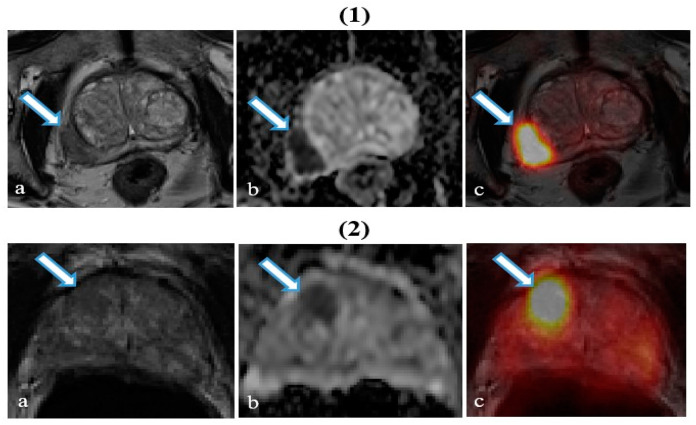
Patients with prostate cancer to the left of the peripheral zone with a Gleason score 4 + 5 = 9 (1) and transitional zone with a Gleason score 3 + 4 = 7 (2). The arrows in the axial section point to areas of cancer. (a) T2-weighted image showing a poorly defined cancerous lesion. (b) ADC map with a corresponding cancer lesion. (c) Overlapped T2-weighted and reconstructed PET images.
